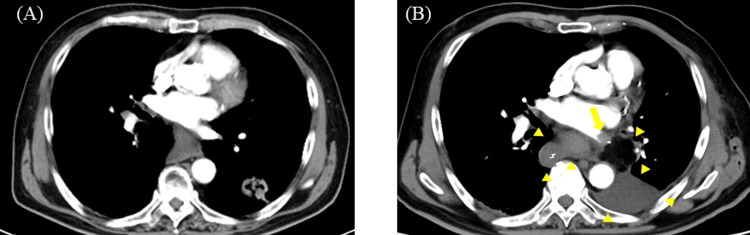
Contrast-enhanced chest computed tomography (CT). (A) Preoperative CT showing a mild hiatal hernia. (B) Post-thrombectomy CT. The left inferior pulmonary vein is compressed by an exacerbated hiatal hernia (arrowheads), and a filling defect is noted within the vein (arrow).
